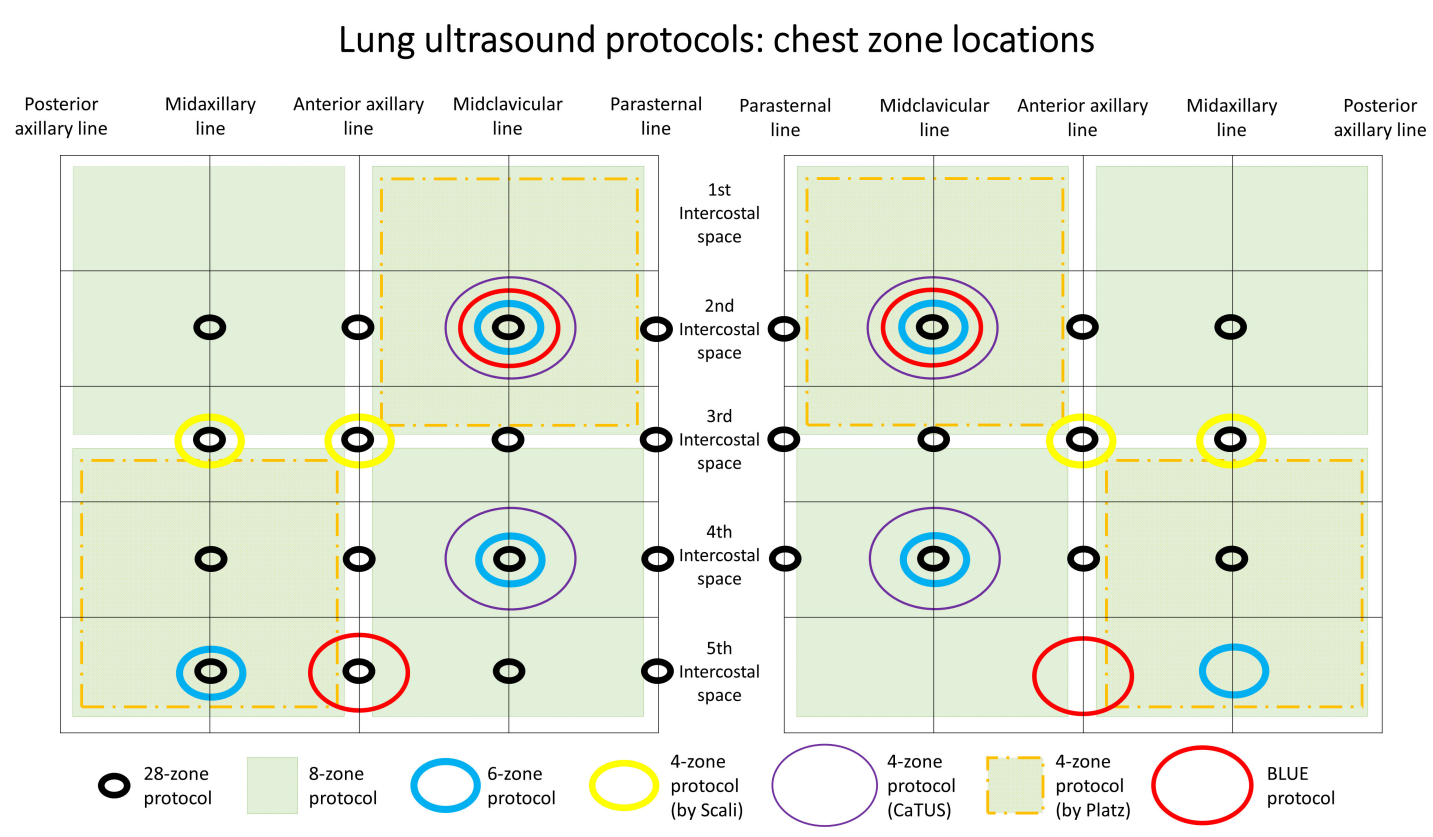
Chest zone locations with different lung ultrasound
